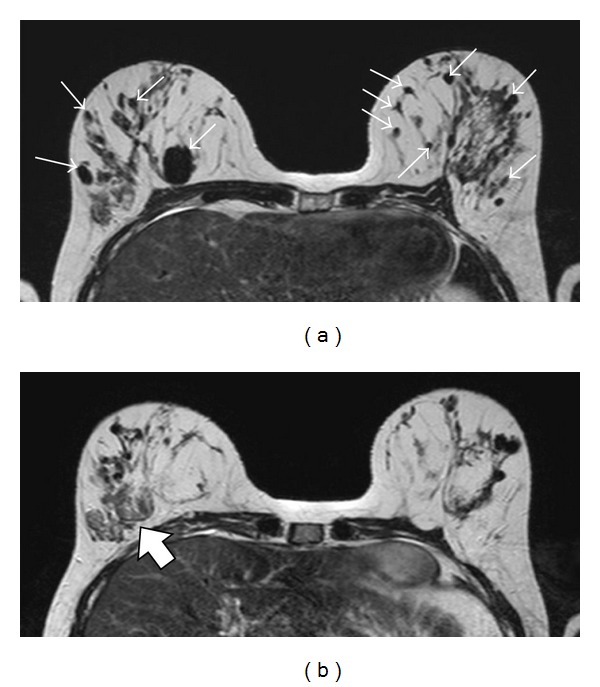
Transverse T2-weighted images show multiple coarse calcifications in both breasts ((a) small arrows) and a large, lobulated mass in the lower lateral quadrant of the right breast ((b) white arrow).
